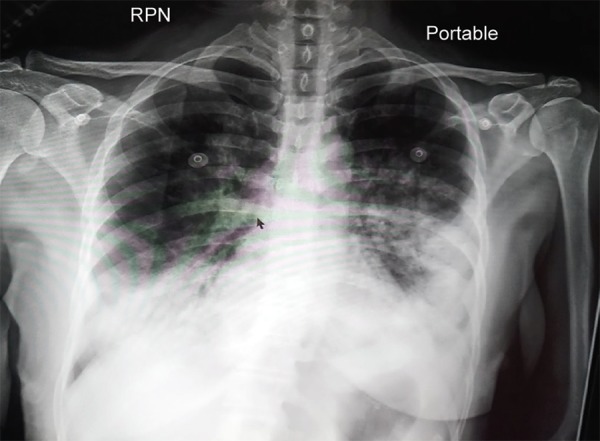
Xray chest on presentation 1 showing bilateral infiltrates
